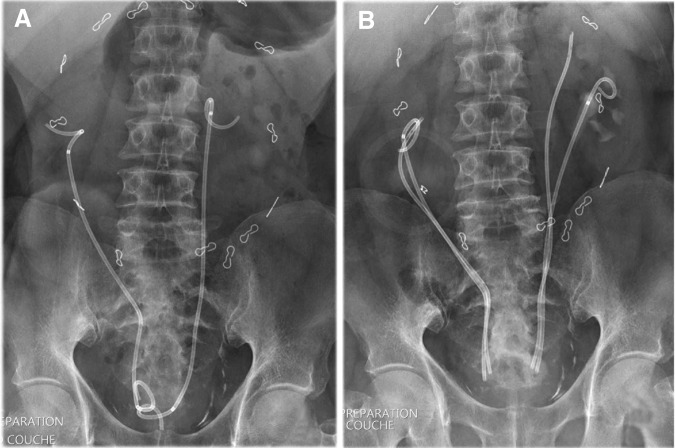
Appearance of ureteral stents on X-ray. (A) Double-pigtail tumor stent with obstruction and incontinence. (B) Sectioned tandem ureteral stents and disappearance of obstruction and incontinence.
